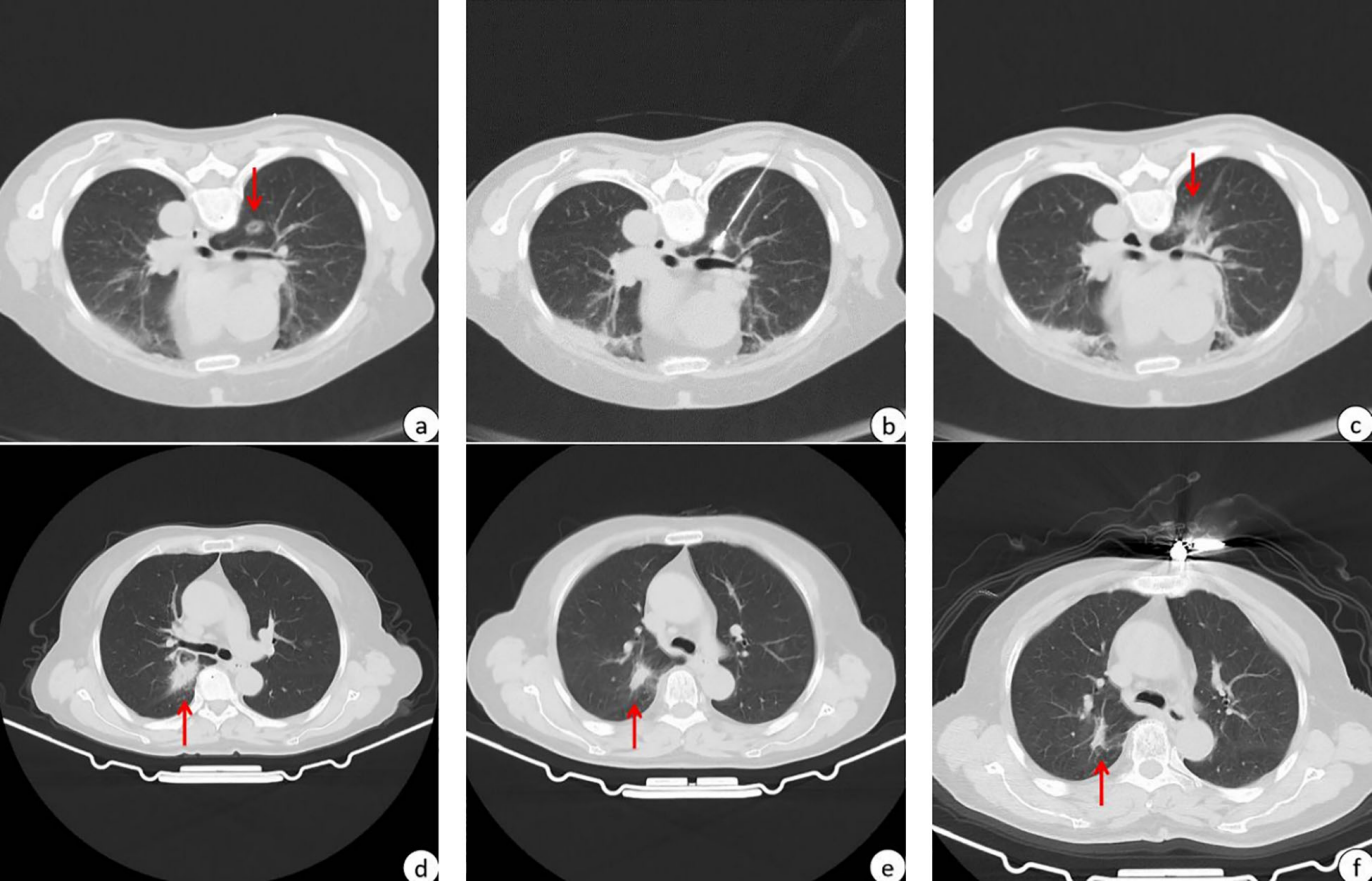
A patient with high-risk pulmonary nodules treated with MWA within 3 months of Omicron infection. The red arrows in the figure point to high-risk pulmonary nodules to be subjected to MWA or post-MWA areas. (A) 13.8*10.7 mm GGN in the upper lobe of the right lung before MWA; (B) CT scan during MWA; (C) after MWA, the halo shadow (36.8*30.2mm) was significantly larger than the lesion; (D) imaging performance 1 months after MWA, and post-ablation area 31.2*18.4mm; (E) imaging performance 4 months after MWA, and post-ablation area 17.9*10.7 mm; (F) imaging performance 10 months after MWA, and post-ablation area 16.7*6.5 mm.
